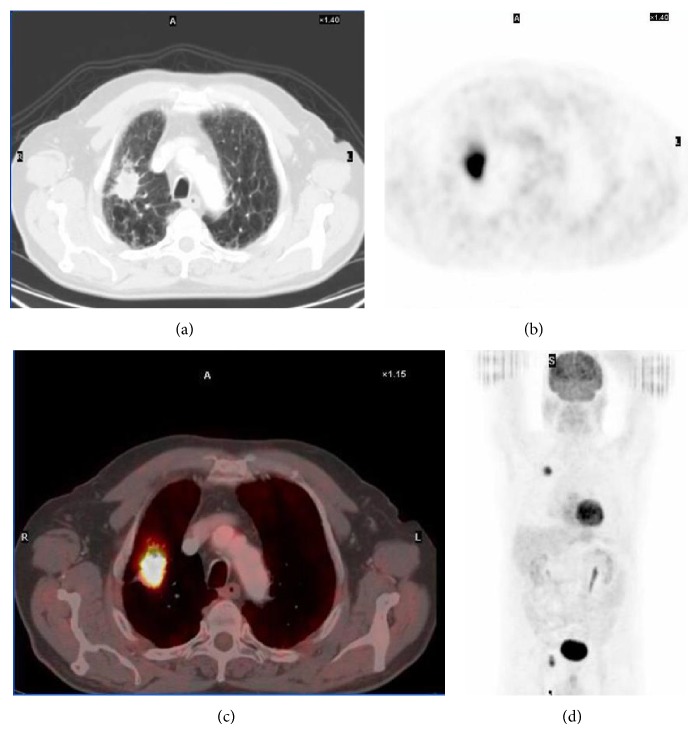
Images for a 60-year-old man. (a) CT image of an NSCLC primary tumor (epidermoid carcinoma) in the right upper lobe. (b) PET image showing intense ^18^F-FDG uptake. (c) PET/CT image showing tumor localisation and radiotracer uptake. (d) Coronal whole-body PET image.
